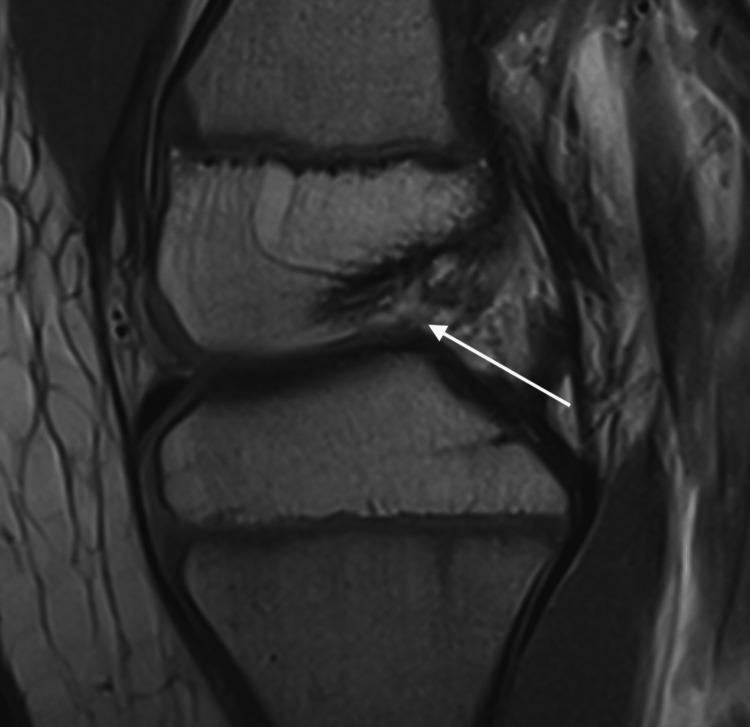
Lateral Right Knee MRI of Patient 1 White arrow: the absence of anterior cruciate ligament
